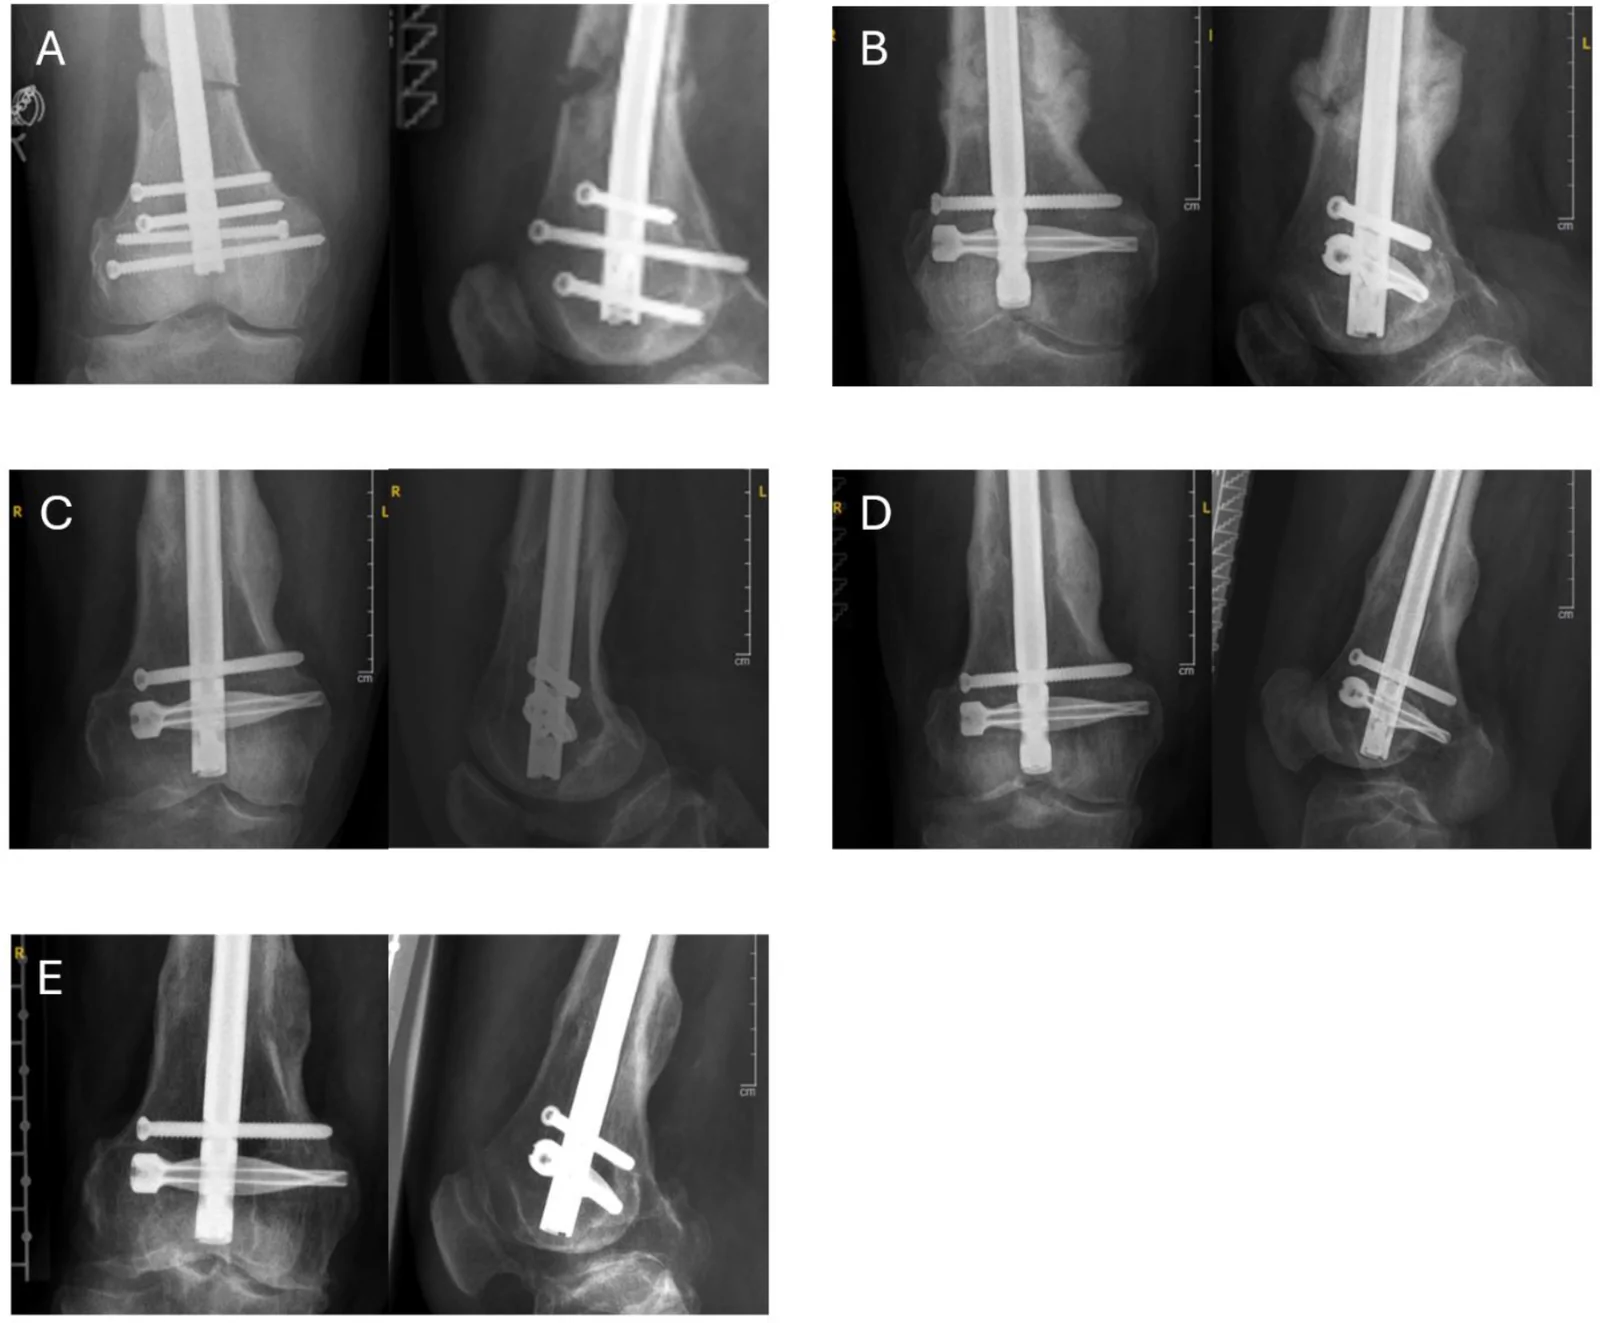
Example 1 of a case with a femur non-union during a 10-year follow-up. (A) Preoperative X-ray (B) Postoperative X-ray after 6 months. (C) Postoperative X-ray after 12 months. (D) Postoperative X-ray after 24 months with full osseus consolidation. (E) Postoperative X-ray after ten years with still ongoing remodeling
